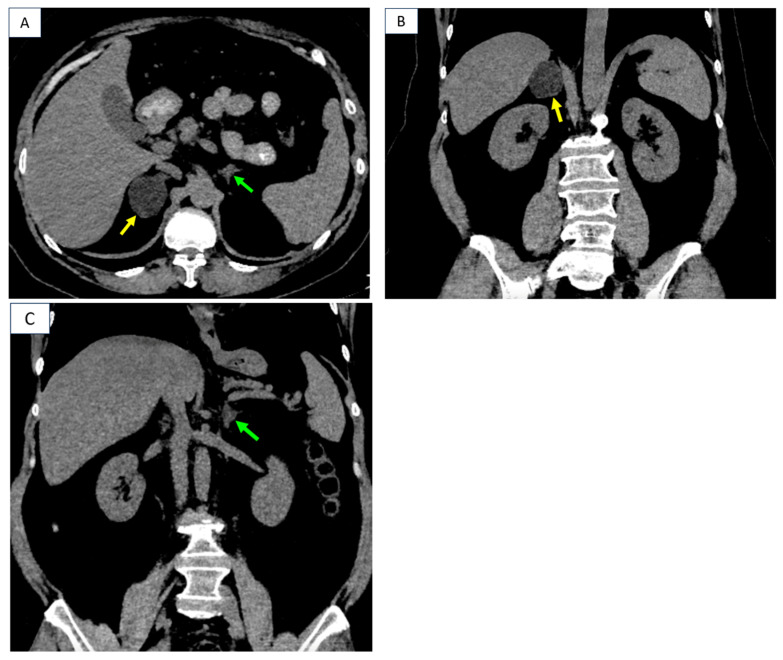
In the meantime, since the age of 56, she was confirmed with arterial hypertension, requiring re-assessment at a tertiary center of endocrinology. The clinical aspects, while not being highly suggestive for a typical Cushing’s syndrome, pointed out metabolic complications in terms of obesity (a body mass index of 36 kg/sqm); dyslipidemia [increased triglycerides of 205 (normal < 200) mg/dL, and total cholesterol of 248 (normal < 200) mg/dL]; impaired glucose tolerance based on a 2 h glycemia during 75 g oral glucose tolerance test of 147 mg/dL, mildly elevated glycated hemoglobin A1c of 6.4 (normal: 4.8–5.9) %, and insulin resistance according to a HOMA-IR (Homeostasis Model Assessment Model of Insulin Resistance) level of 5.3 (insulin resistance at HOMA-IR above the cutoff 2). Hormonal panel was conclusive for Adrenocorticotropic Hormone (ACTH)-independent (autonomous) cortisol excess based on: suppressed baseline morning ACTH of 2.7 (normal: 7.2–63.3) pg/mL, normal morning plasma cortisol of 13.1 (normal: 4.82–19.5) µg/dL without suppression at 1 mg overnight dexamethasone testing according to the second-day plasma cortisol level of 13.9 (normal < 1.8) µg/dL. The central Dual-Energy X-Ray Absorptiometry (DXA) scan excluded osteoporosis/osteopenia (lowest T-score of +0.8 SD, and bone mineral density of 1.098 g/sqcm at total hip), and the trabecular bone score of 1.388 was normal. CT showed bilateral adrenal tumors [3. CT scan (native phase): right adrenal tumor (yellow arrow) of 3.7 by 4.4 by 4.7 cm with negative density, heterogeneous, in close contact with the inferior vena cava, the upper pole of the right kidney, the fifth hepatic segment and the right diaphragmatic crus and left adrenal tumor of 1.1 by 1.6 cm (green arrow); (A) axial plane; (B) coronal plane; (C) coronal plane].
